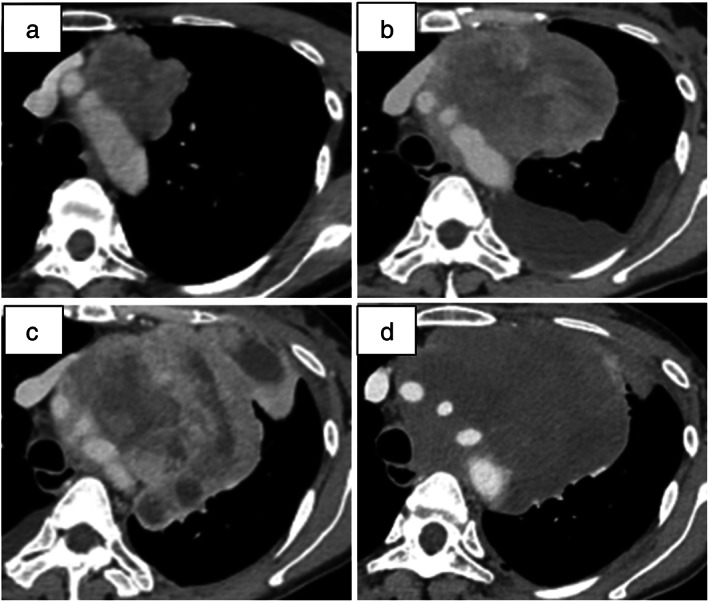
Contrast‐enhanced chest computed tomography images. Images showing the (a) anterior mediastinal tumor at the initial visit to our hospital and (b) two months after the first visit to our hospital, at first‐line chemotherapy initiation. (c) Image showing an aggressively increased tumor at the start of pazopanib treatment. (d) Image showing tumor size reduction and tumor contrast effect at 3 months after pazopanib initiation
